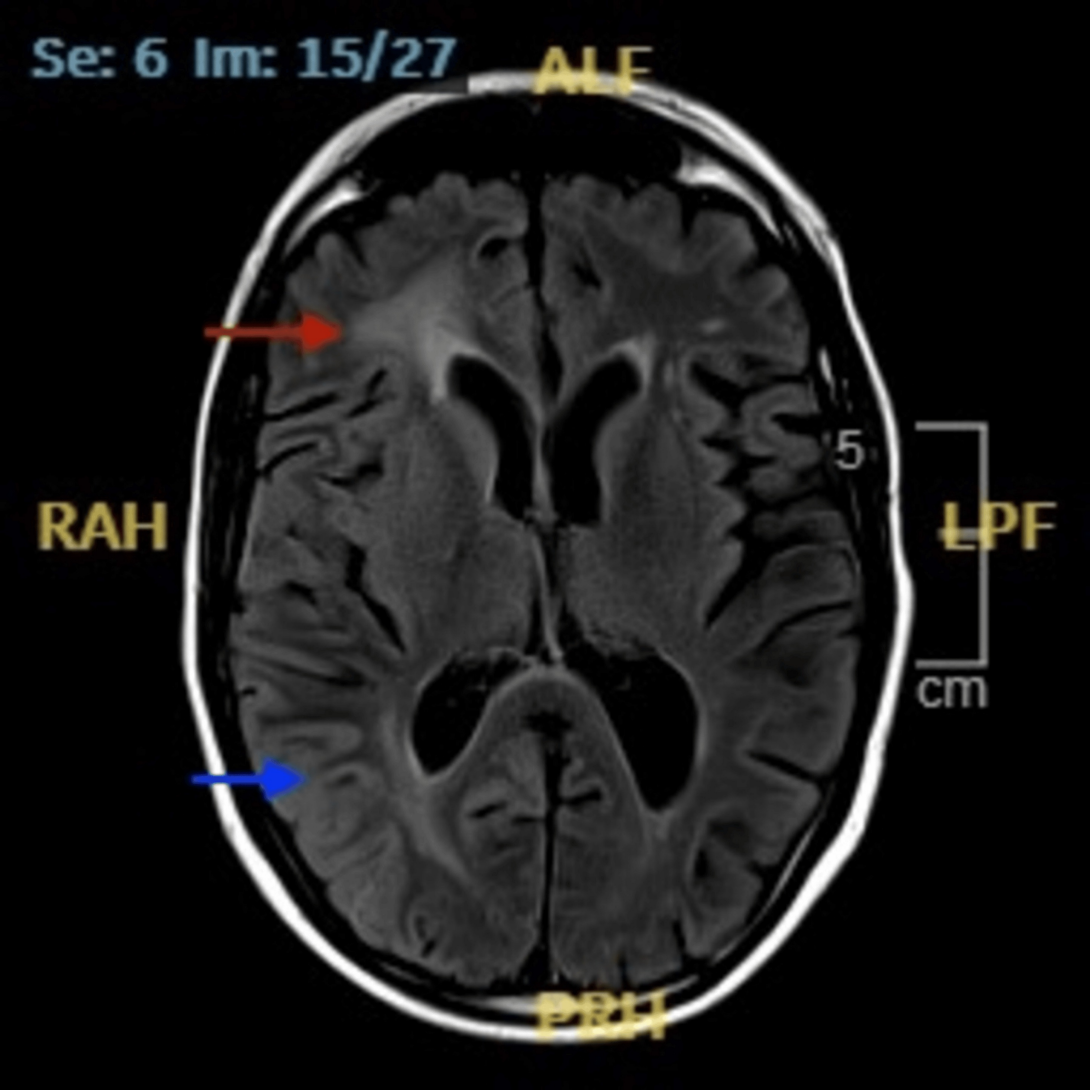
MRI brain (T2/FLAIR) Red arrow: small vessel ischemic changes. Blue arrow: mild gyral enhancement as compared to the left side MRI: magnetic resonance imaging; FLAIR: fluid attenuated inversion recovery
